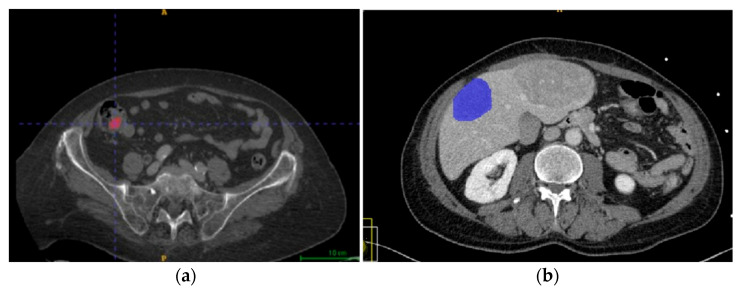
(a) Segmentation of the primary ileal NET in portal venous phase CT images, displayed in the axial plane. The segmented region of interest (ROI) corresponds to the primary ileal neoplasms and it is highlighted in red. (b) Segmentation of a liver metastasis from an ileal NET on portal venous phase CT images, displayed in the axial plane. The segmented ROI is highlighted in blue.
